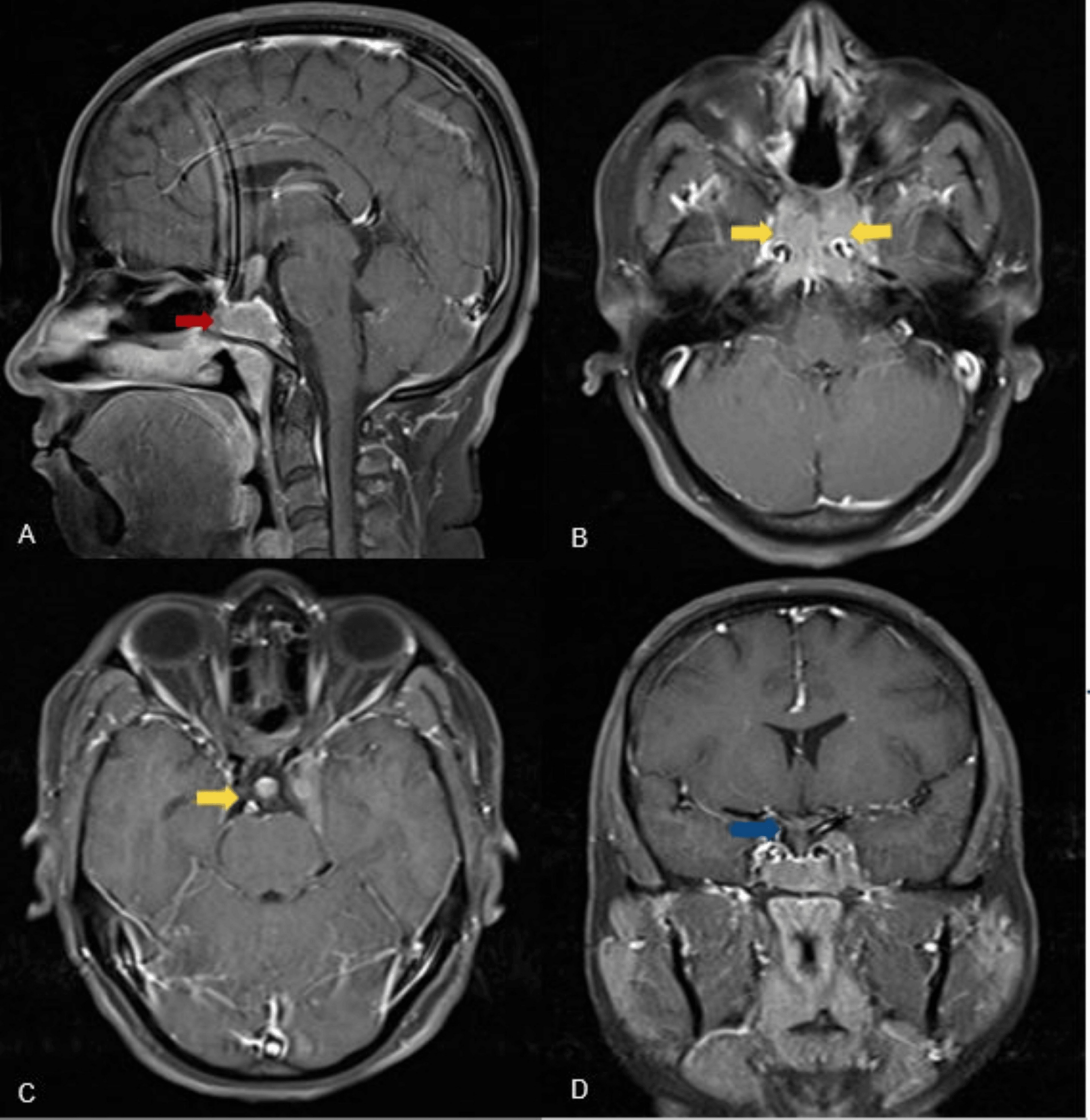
MRI findings - 2 Post-contrast T1 fat saturation sequences showing pituitary macroadenoma showing near-homogeneous post-contrast enhancement (red arrow), and thickening and enhancement of infundibulum (yellow arrows) with extension into bilateral cavernous sinuses (blue arrow). A: sagittal; B and C: axial; D: coronal MRI: magnetic resonance imaging
